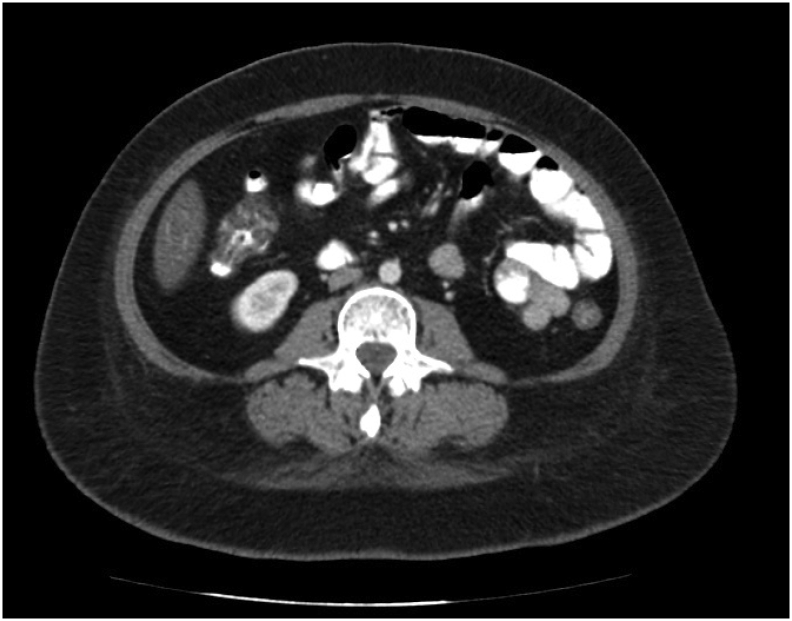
Abdominopelvic CT scan with intravenous and oral contrast agent.
